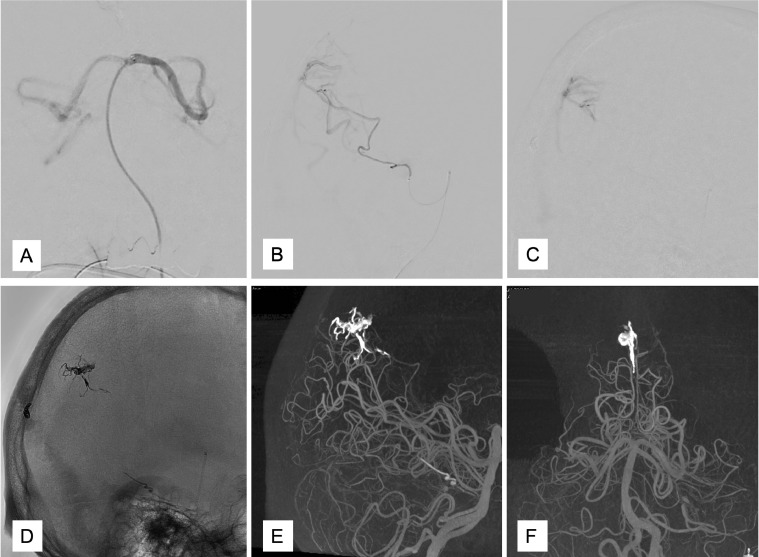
GuidePost was placed in P1 of the left posterior cerebral artery (A). The Marathon catheter was navigated into the artery of Davidoff and Schechter (B). The Marathon reached the shunt point (C). Spot lateral radiograph of the skull showing the Onyx cast, following transarterial embolization (TAE) (D). The minimum intensity projection image observed after left vertebral artery injections and TAE showing no residual early venous filling, with an Onyx cast filling the cortical vein (E, F). (E) lateral view, (F) anterolateral view
